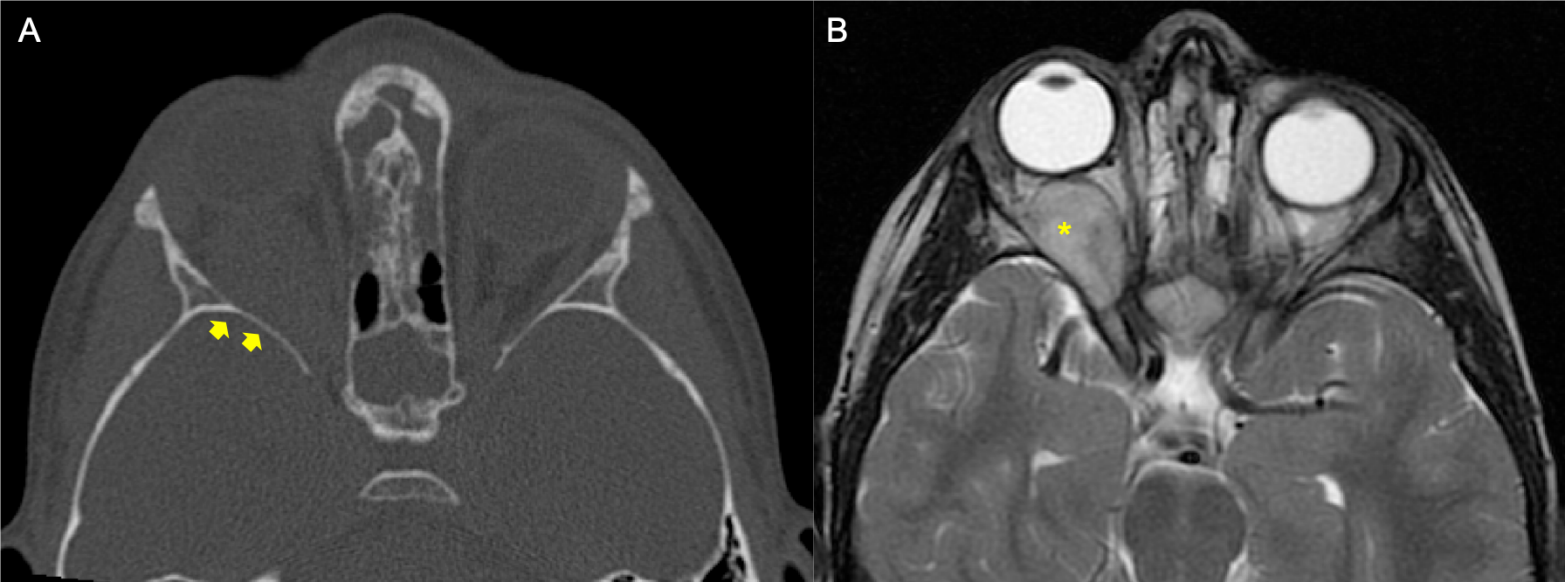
Example of a patient affected by rhabdomyosarcoma (RMS) with no bone erosion (BE). The patient underwent orbital CT scan and MRI at staging. (A) Bone window axial reconstruction of the CT did not show BE (*yellow arrows*). (B) Axial T2-weighted image of magnetic resonance confirmed right-side orbital mass (*yellow asterisk*).
